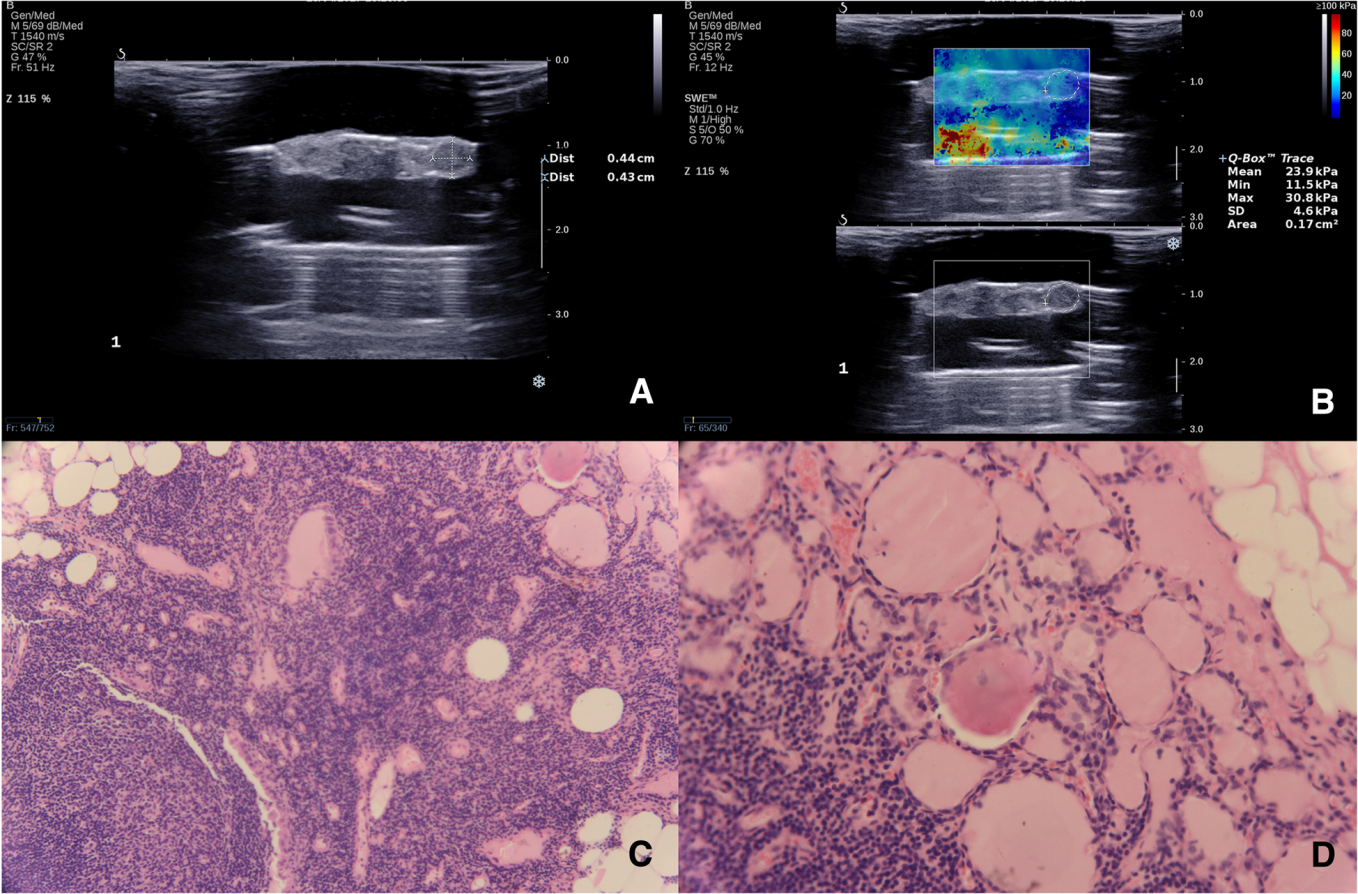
A 61-year-old man with papillary thyroid carcinoma in the right lobe of thyroid. The long diameter and short diameter of one lymph node at ipsilateral level VI were 4.4 mm and 4.3 mm (a). S/L ratio was 0.977. E_mean_, E_max_ and E_SD_ were 23.9 kPa, 30.8 kPa and 4.6 kPa respectively (b). Gray-ultrasound score and combination score were 2 and 5 respectively. This lymph node was confirmed to be metastatic by pathological diagnosis (c, original magnification × 40, and d, original magnification × 100)
